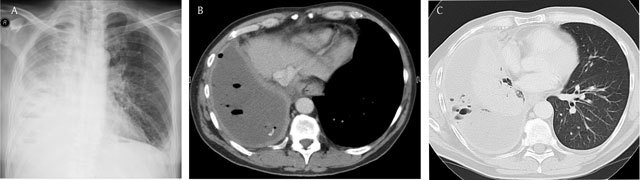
Empyema in a patient with cough and fever since 1 week – Chest radiograph (a) demonstrates a hazy increased opacity with multiple air bubbles overlying the lower lung. An axial contrast-enhanced CT view in mediastinal (b) and lung window (c) at the level of the lung base shows thickening and an enhancement of the parietal pleura and the visceral pleura (split pleura sign). Note the multiple air bubbles in the empyema.
